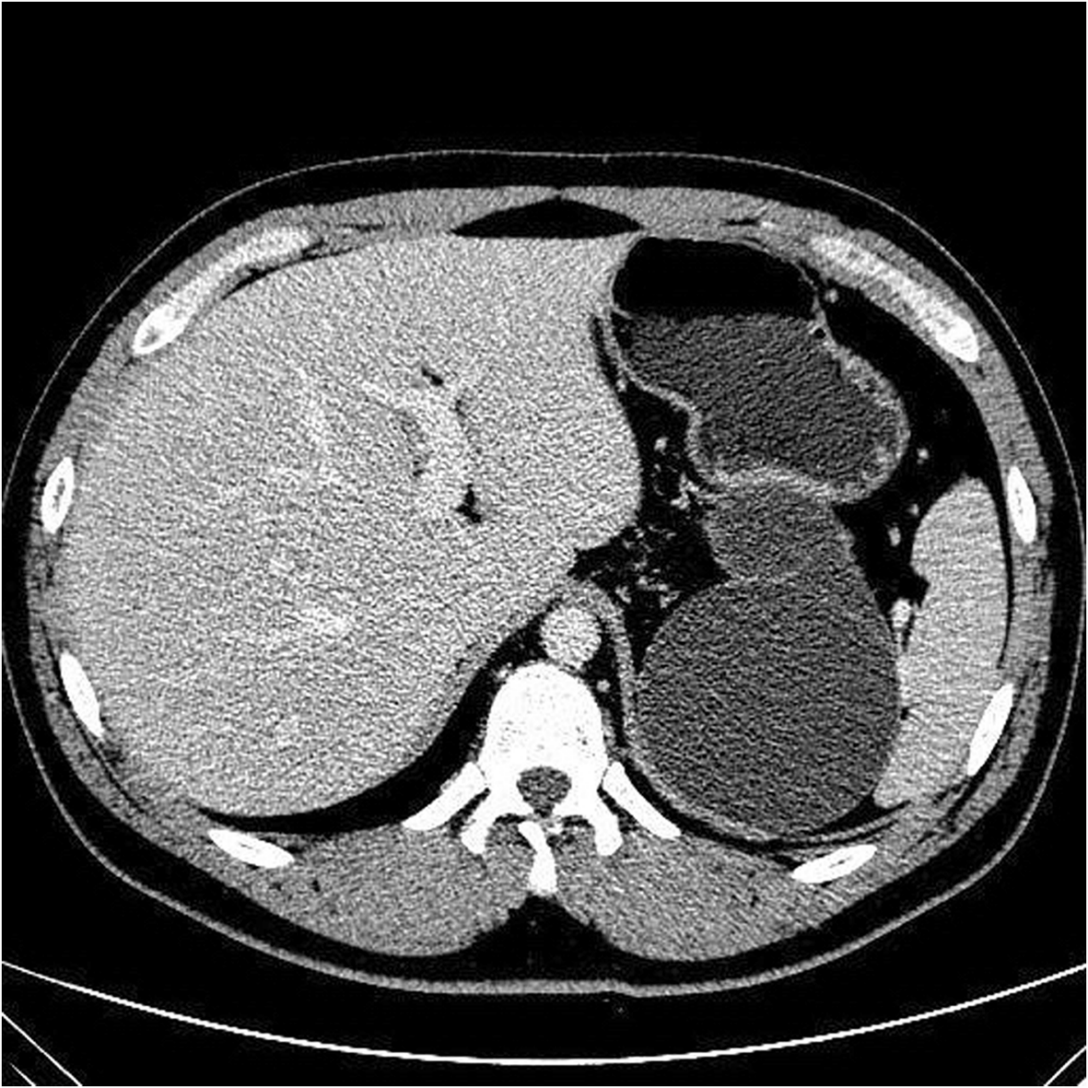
Computed tomography of the abdomen (with contrast agent). A well-defined oval lesion is seen immediately adjacent to the gastric corpus and left adrenal gland area. A linear septum can also be seen.
